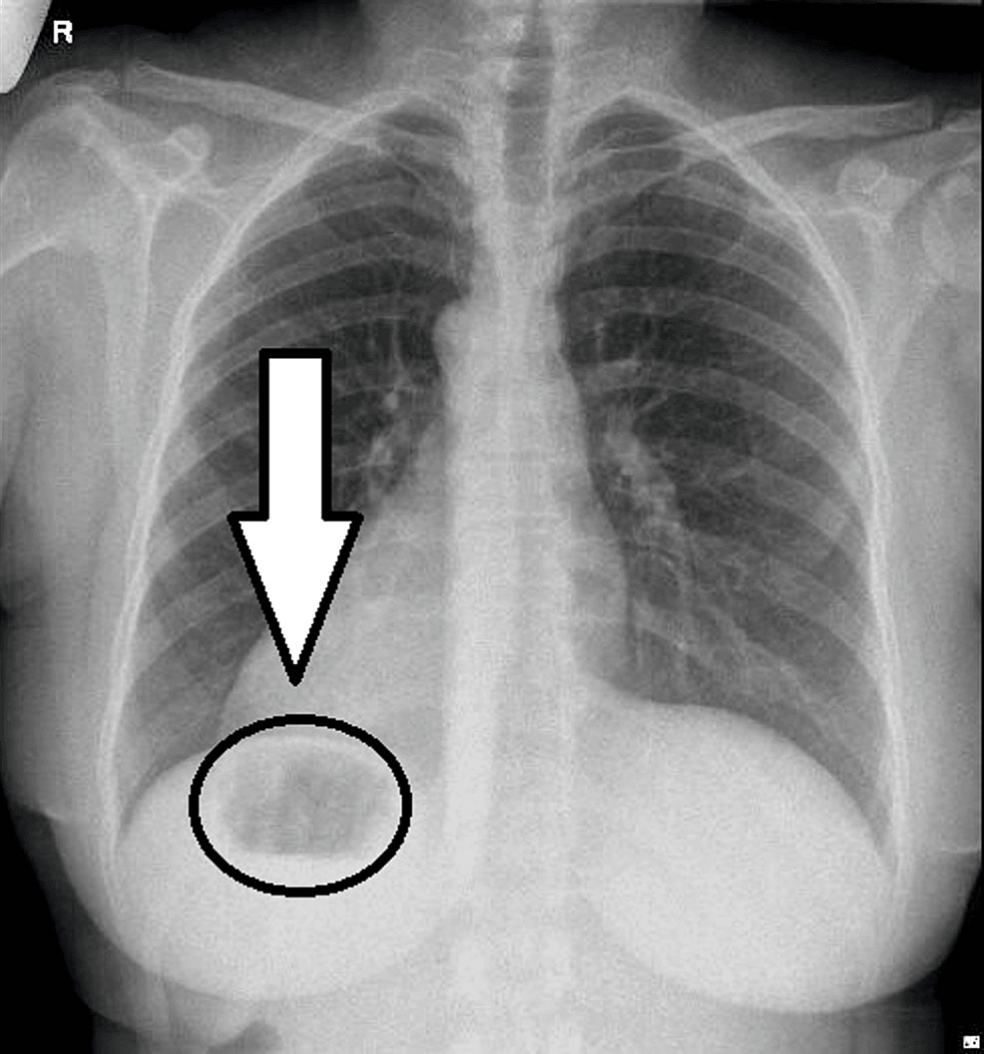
Posterior-anterior chest X-ray. The right-sided cardiac apex (shown by the arrow). Gastric gas under the right diaphragm (black circle).
